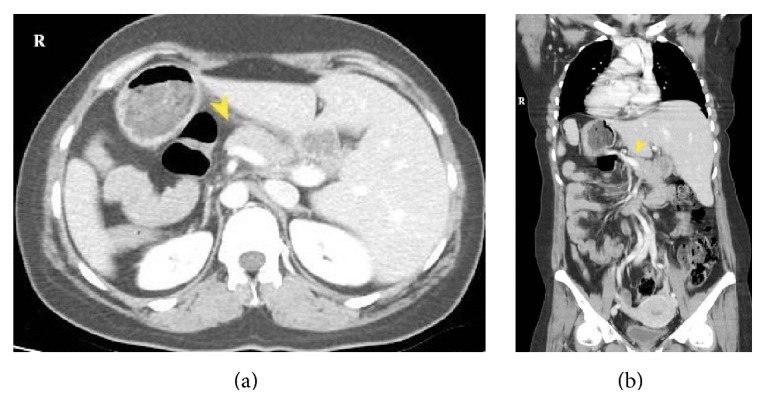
Abdomen CT; pancreatic tissue ends by bounds of superior mesenteric vein axis.
